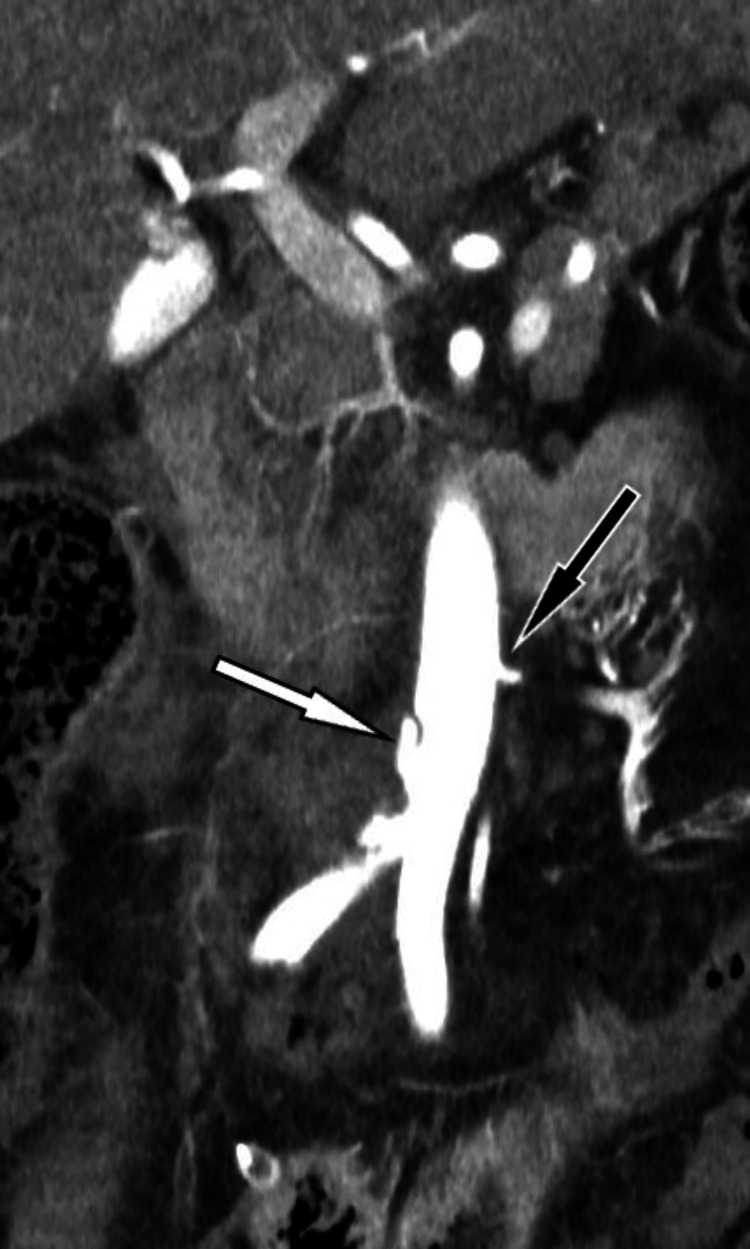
Computed tomography angiography after surgery, coronal image, dissection of the distal part of the abdominal aorta (white arrow) and the left aberrant renal artery (black arrow)
